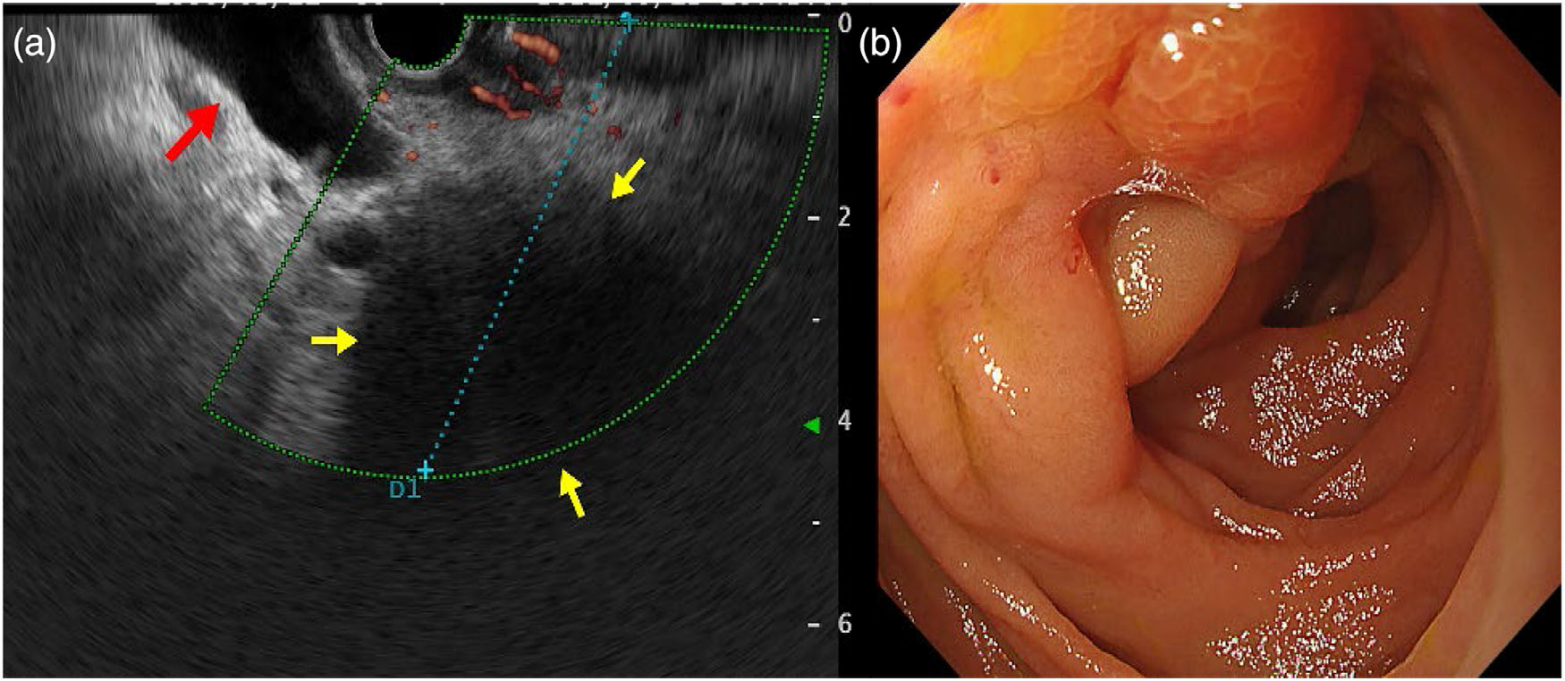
Endoscopic ultrasonography and upper endoscopy images. (a) Endoscopic ultrasonography images. A hypoechoic mass of 40 mm in size is seen in the pancreatic head (yellow arrow), and the common bile duct is disrupted at that site and dilated upstream (red arrow). (b) Upper endoscopy shows stenosis with mucosal irregularity on the anal side of the Vater papilla. A biopsy was performed at the stenotic site, and the pathological diagnosis was adenocarcinoma
